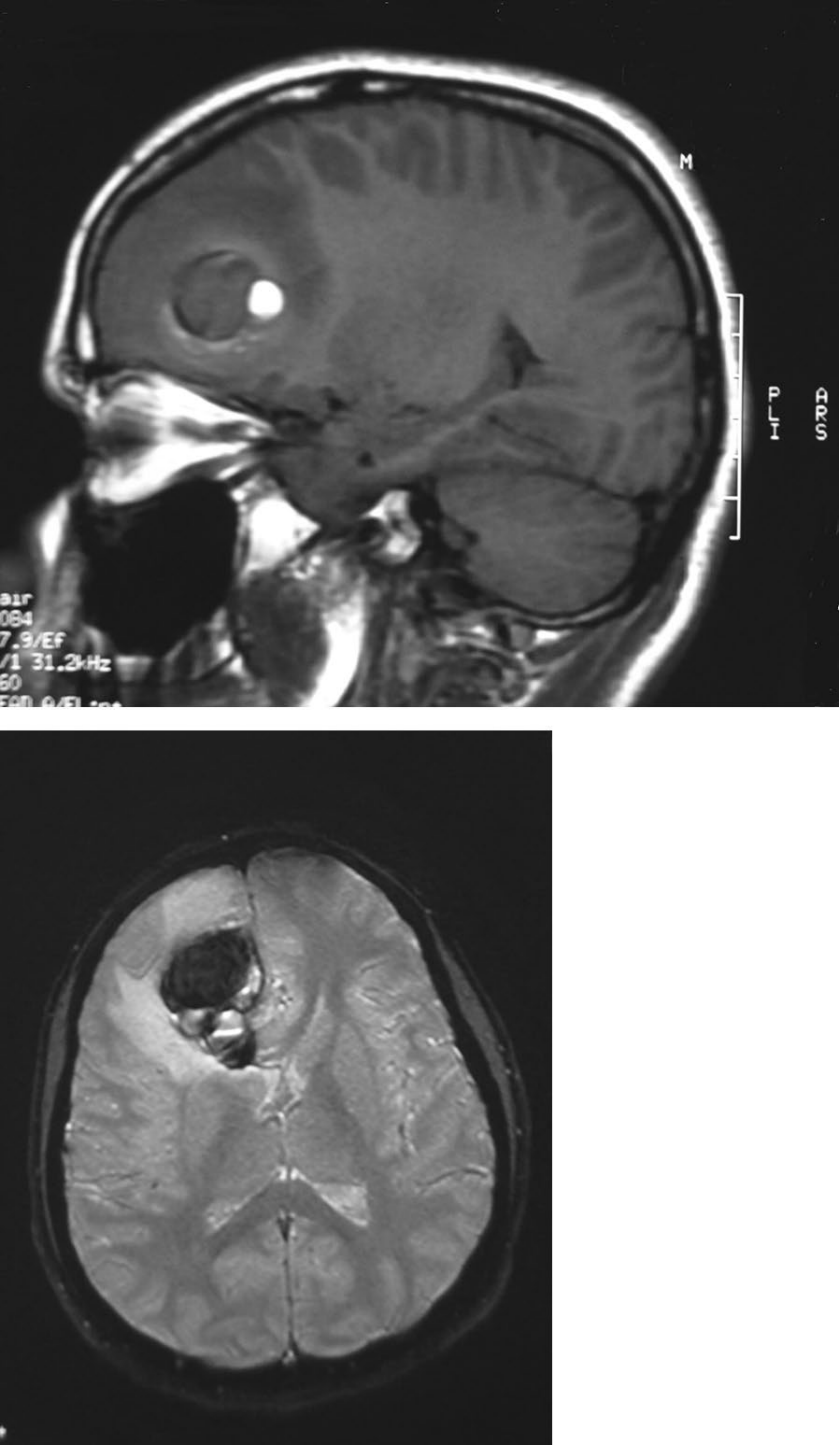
Magnetic resonance imaging showing a new contrast enhancing mass in the frontal lobe at the site of prior abscess.
